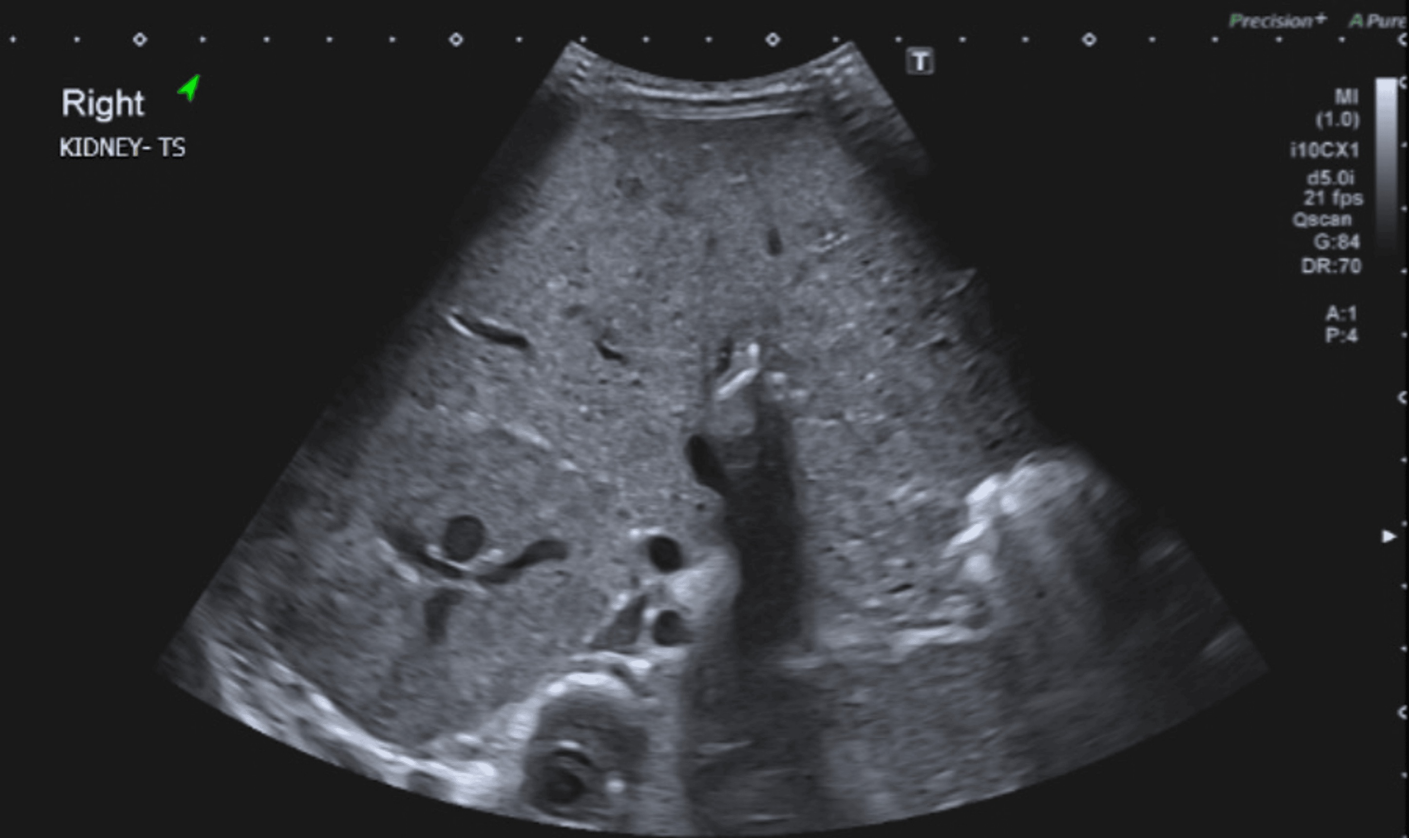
Abdominal ultrasonography, transverse cut through right kidney demonstrating loss of corticomedullary differentiation
